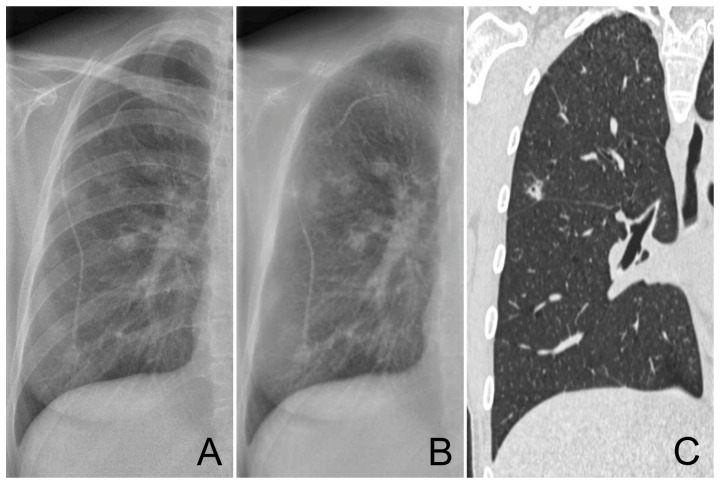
Example of a moderately subtle case of a 35 year old female with multiple focal lesions in the right lung. Without BSI 6 of the 7 observers marked this case with an average suspiciousness score of 63. With BSI all observers marked the case with an average suspiciousness score of 91. Original radiograph (A), bone suppressed image (B), coronal CT slice (C).
